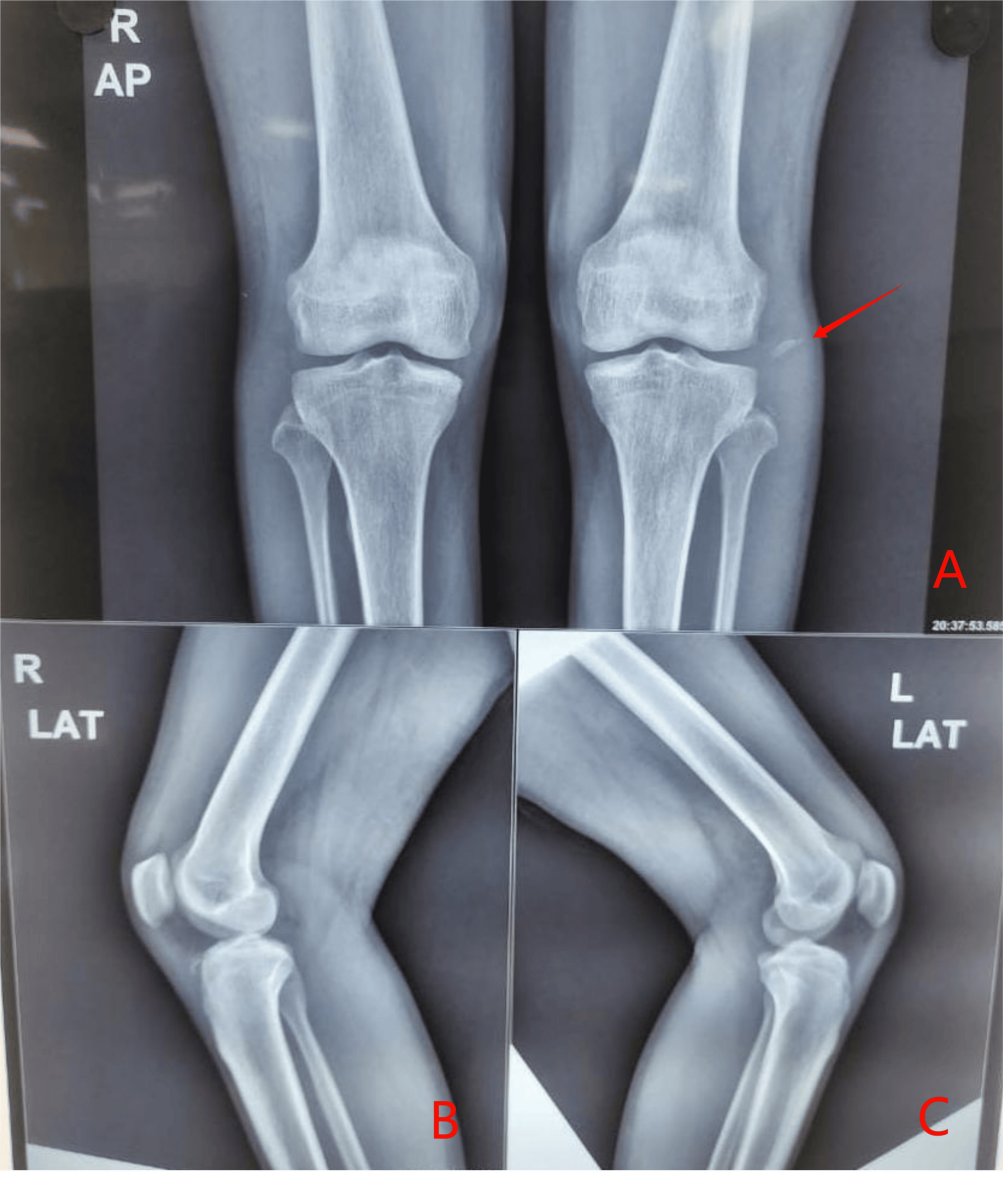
Preoperative X-ray (antero-posterior and lateral views of both knees) (A) Antero-posterior view of both knees with the arrow showing fibular head avulsion fracture in the left knee. (B and C) Lateral view of both the right and left knee
